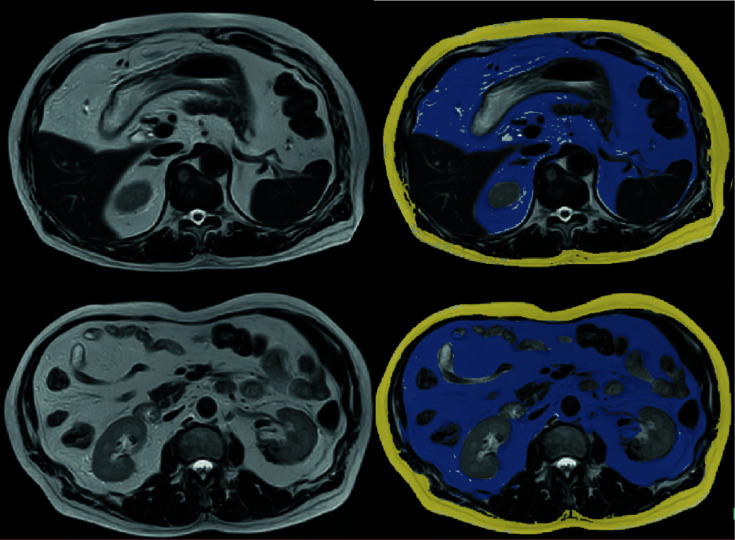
Representative examples of MRI scans with segmentation of visceral adipose tissue (blue) and subcutaneous adipose tissue (yellow).
